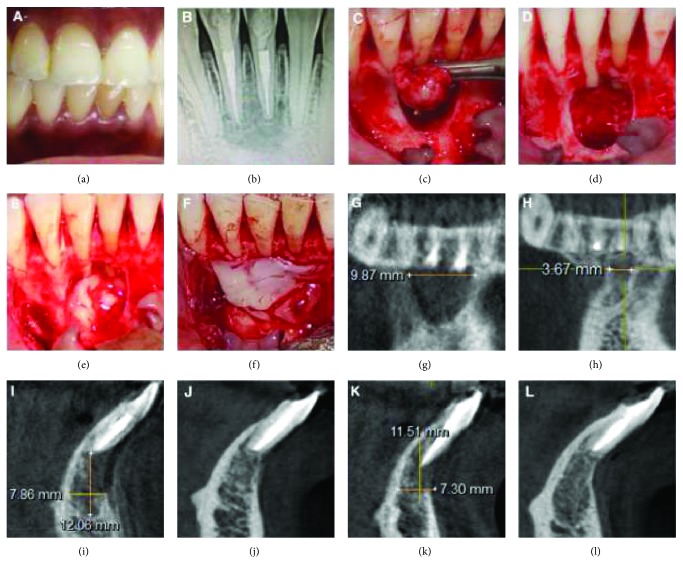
Preoperative clinical and surgical procedure and radiographic images: IOPA and CBCT images (Case Report 2). (a) Pre-op clinical photograph shows discoloured 31. (b) Preoperative IOPA showing the periapical lesion involving the apices of 11 and 12 and extending to the mesial margin of 13. (c) Complete curettage of the lesion. (d) Apicectomy done and MTA retrograde filling given in 31 and 41. (e) CGF fibrin-rich block placed in the bony defect. (f) CGF membrane placed before suturing of the flap. (g) Pre-op CBCT image showing the lesion measurement in the coronal slice. (h) Post-op CBCT image showing the lesion measurement in coronal slice. (i) Pre-op CBCT image showing the lesion measurement in sagittal slice corresponding to 41. (j) Post-op CBCT image showing the lesion measurement in sagittal slice corresponding to 41. (k) Pre-op CBCT image showing the lesion measurement in sagittal slice corresponding to 31. (l) Post-op CBCT image showing the lesion measurement in sagittal slice corresponding to 31.
